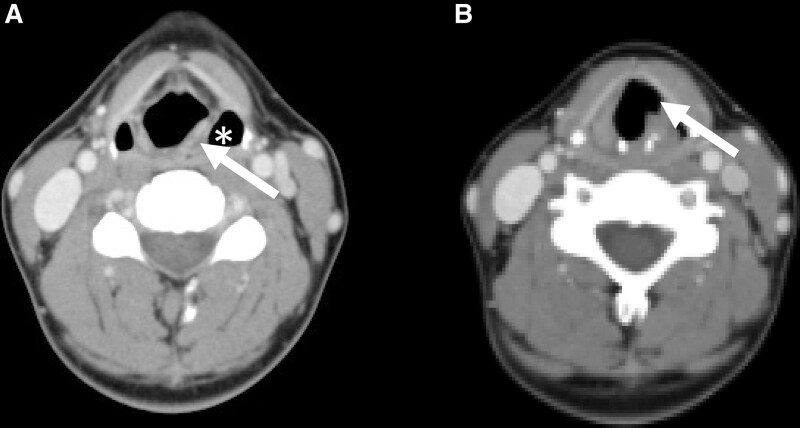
(A) Axial section of neck computed tomography image revealing dilatation of the left pyriform sinus (asterisk) and medialization of the left aryepiglottic fold (arrow). (B) Axial section of neck computed tomography image at the level of the vocal cord revealing dilatation of the laryngeal ventricle, also known as the sail sign (arrow). These features are consistent with left vocal cord paralysis.
